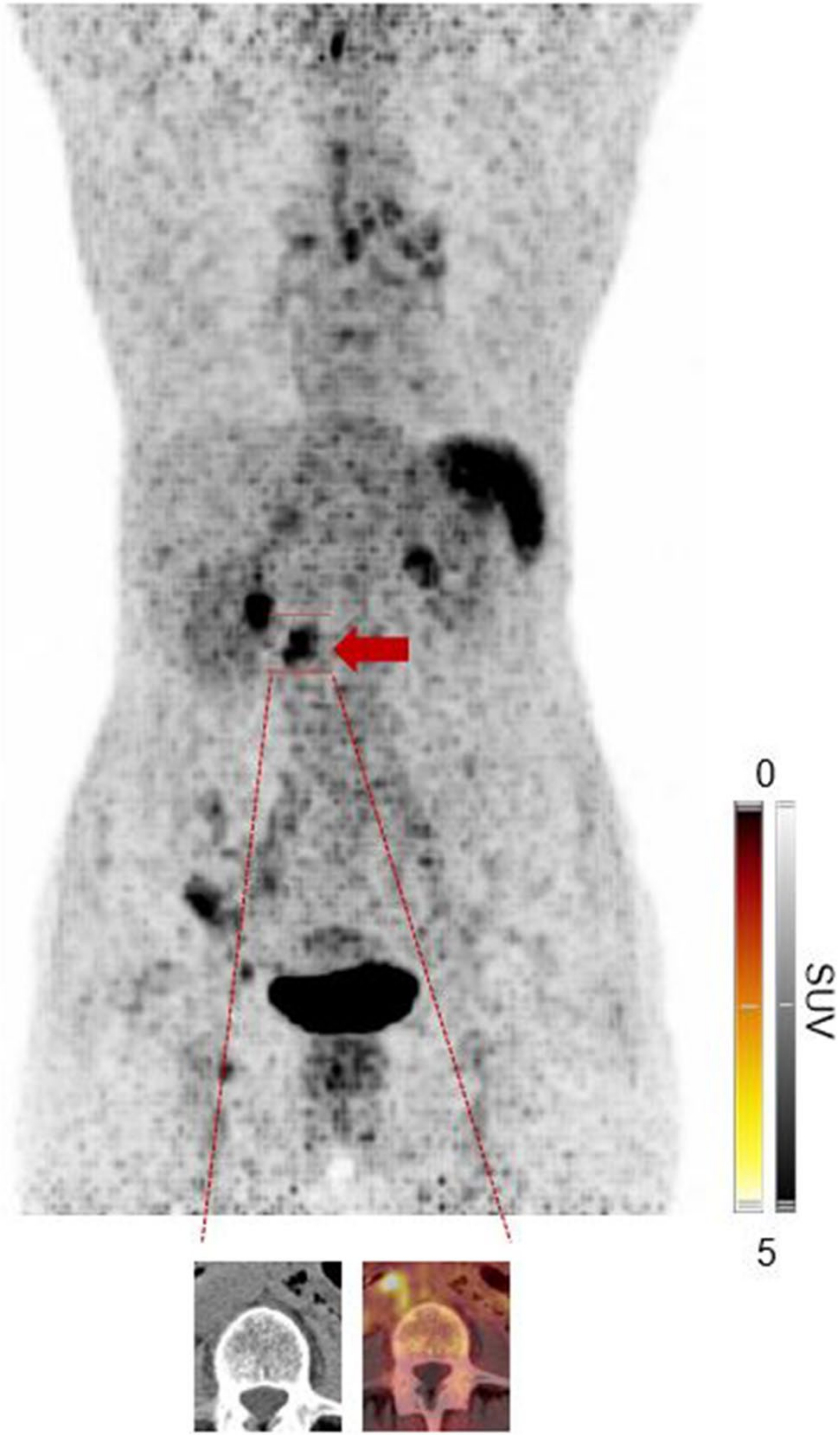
66-year old subject diagnosed with nodal marginal zone lymphoma. As seen on the maximum intensity projection, there were nodal lymphoma manifestations above and below the diaphragm, along with a CXCR4-positive lymphoma manifestation in the intestine (transaxial CT and PET/CT on the bottom). This extranodal involvement was only identified by the experienced, but not by the less experienced readers, highlighting the challenge of interpreting manifestations outside of the nodal system using CXCR4-targeted PET/CT.
